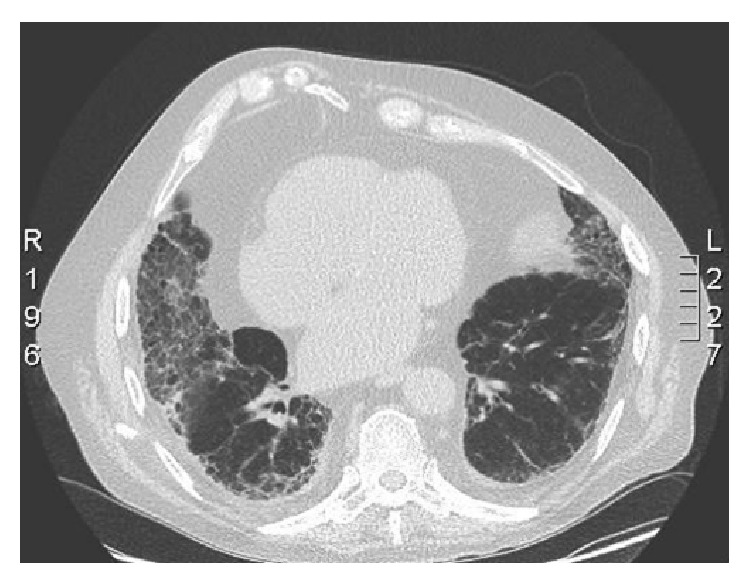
CT scan (prior to treatment) showing diffuse pulmonary fibrosis (honeycombing) and bilateral pleural fluid collection.
